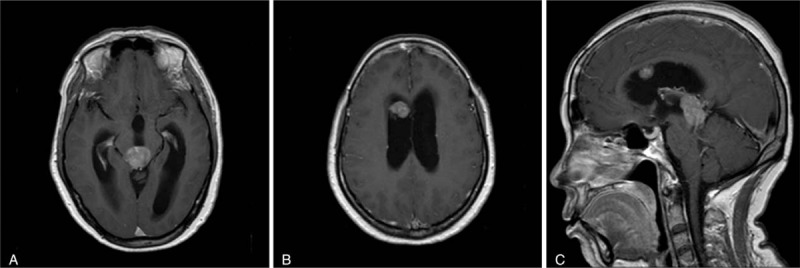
Preoperative contrast T1-weighted MRI showing enhancing lesions in the pineal region (A) and right lateral ventricle (B). Both lesions were visible via sagittal view (C). The lesion demonstrated a heterogeneous enhancement pattern.
